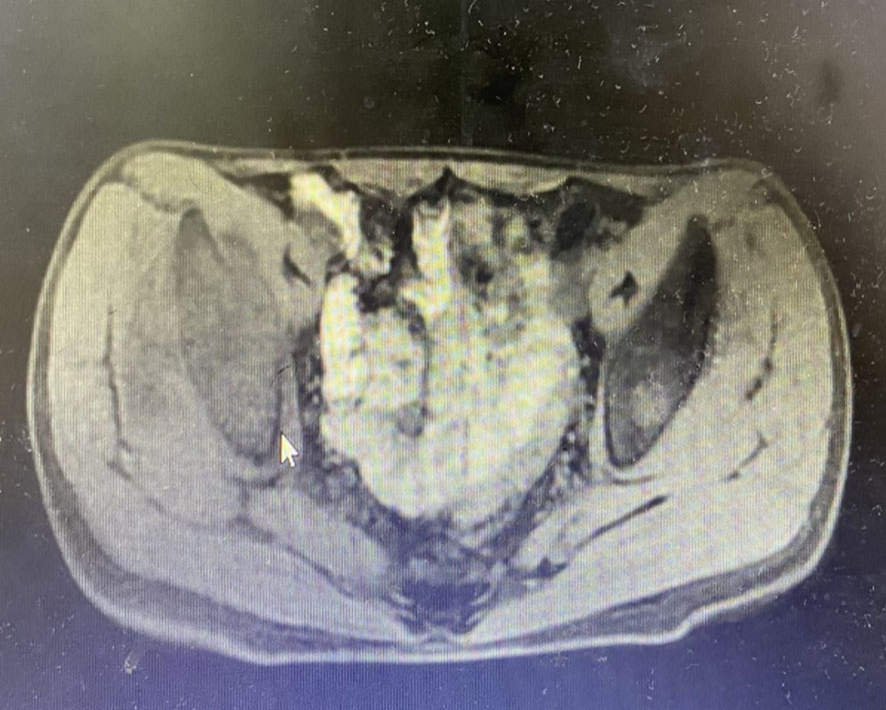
CT imaging characteristics. A 9.1×7.8 cm heterogeneously enhancing retroperitoneal mass adjacent to the left kidney.
